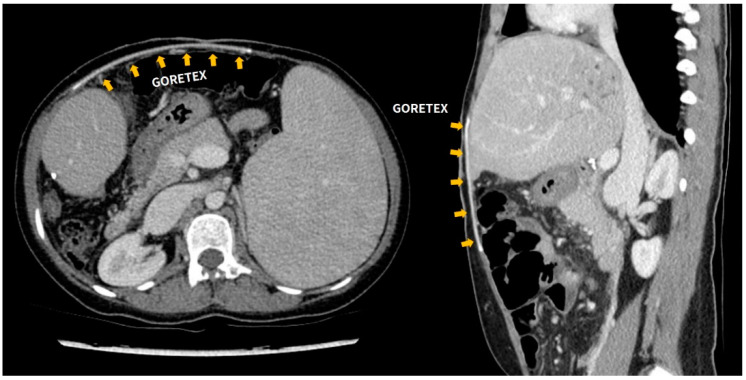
Follow-up abdominal CT scan of patient number 10. Expanded polytetrafluoroethylene (ePTFE-Gore-Tex) graft located between the skin and fascial layer.
